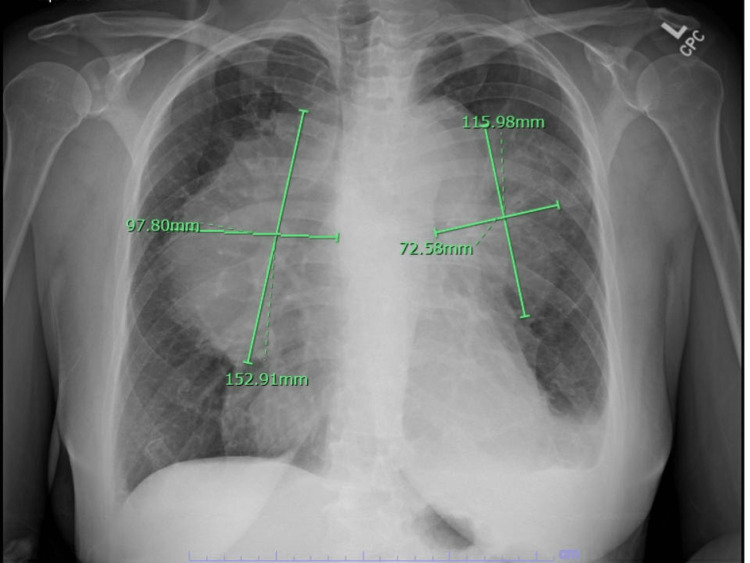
Chest X-ray showed bilateral perihilar masses measuring up to approximately 15.3 x 9.8 cm on the right and 11.6 x 7.2 cm on the left.
